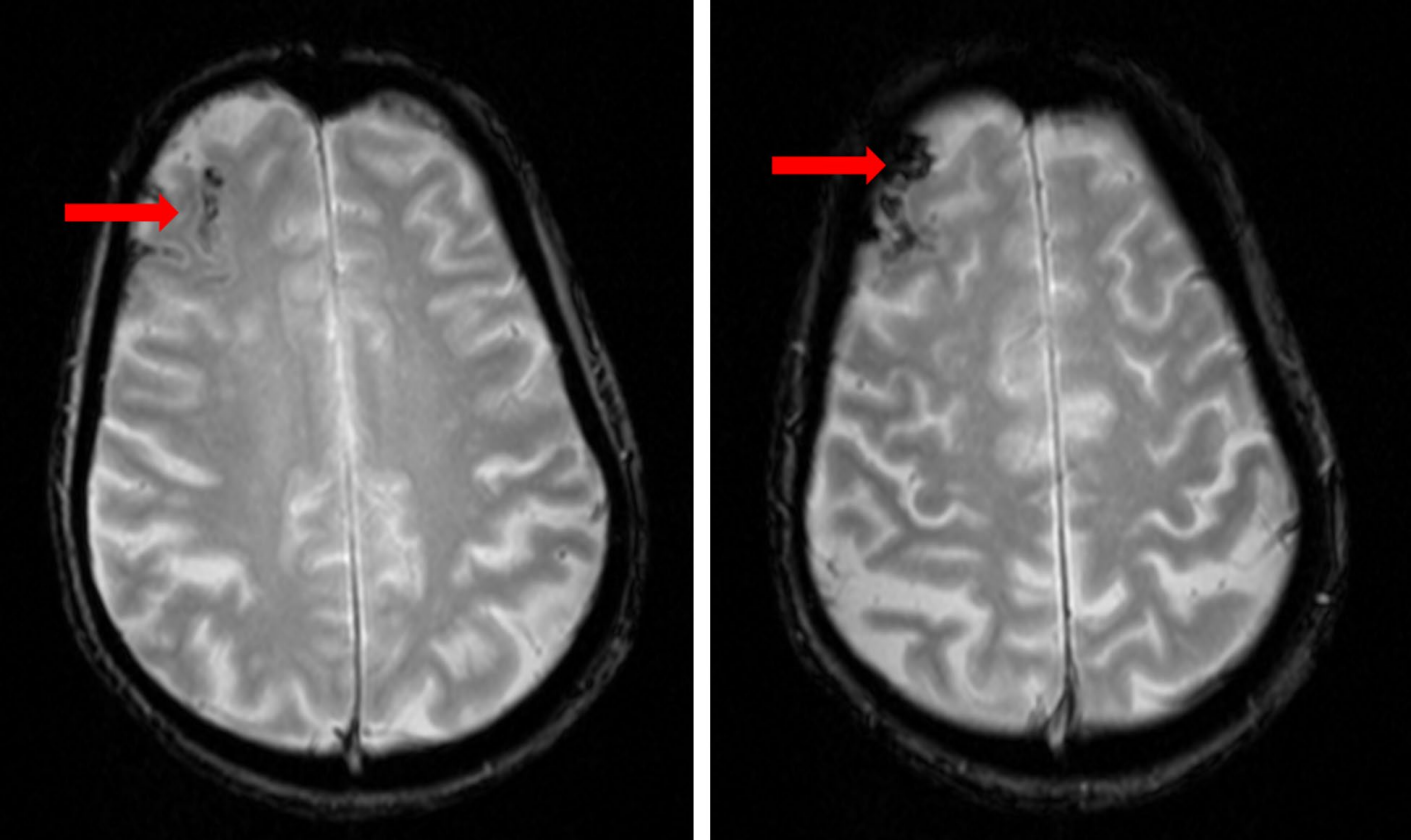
MRI of the brain (axial sequence) gradient recall echo (GRE) image shows hypointense foci in the right frontal region consistent with hemorrhage (*arrows*) in the same patient mentioned in Fig. 1
